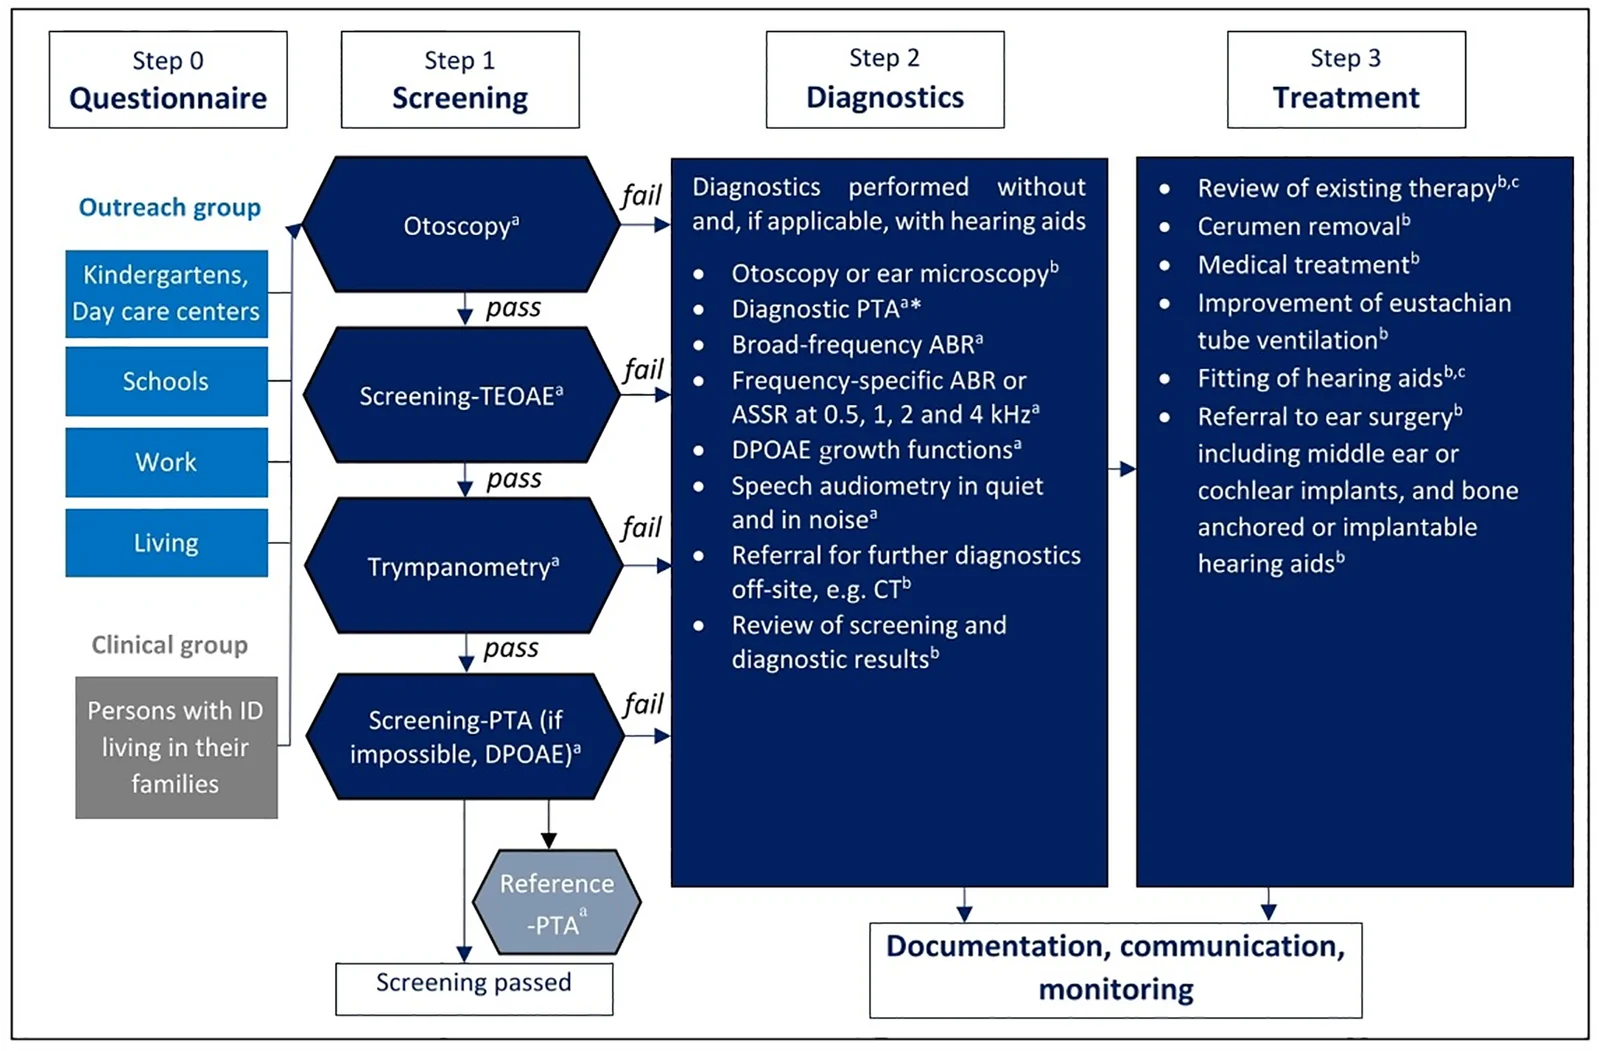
Components of the *HörGeist* screening, diagnostic, and intervention program. ^a^conducted by screening staff; ^b^conducted by physician; ^c^conducted by hearing aid specialist; ^*^In cases of failed screening, reference PTA served as the diagnostic PTA. TEOAE, transient-evoked otoacoustic emissions; PTA, pure-tone audiometry; DPOAE, distortion product otoacoustic emissions; ABR, auditory brainstem response; ASSR, auditory steady-state response; CT, computer tomography (from [35] and modified according to [83]).
